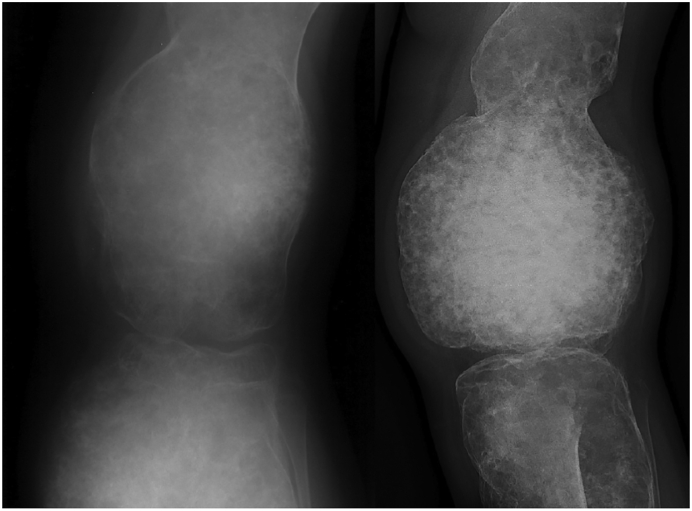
Progression of the lesion in the distal femur of case 1 prior to denosumab therapy on plain X-ray. Left: 1994. Right: 2015. Measurements could not be compared because left X-ray was not calibrated.
